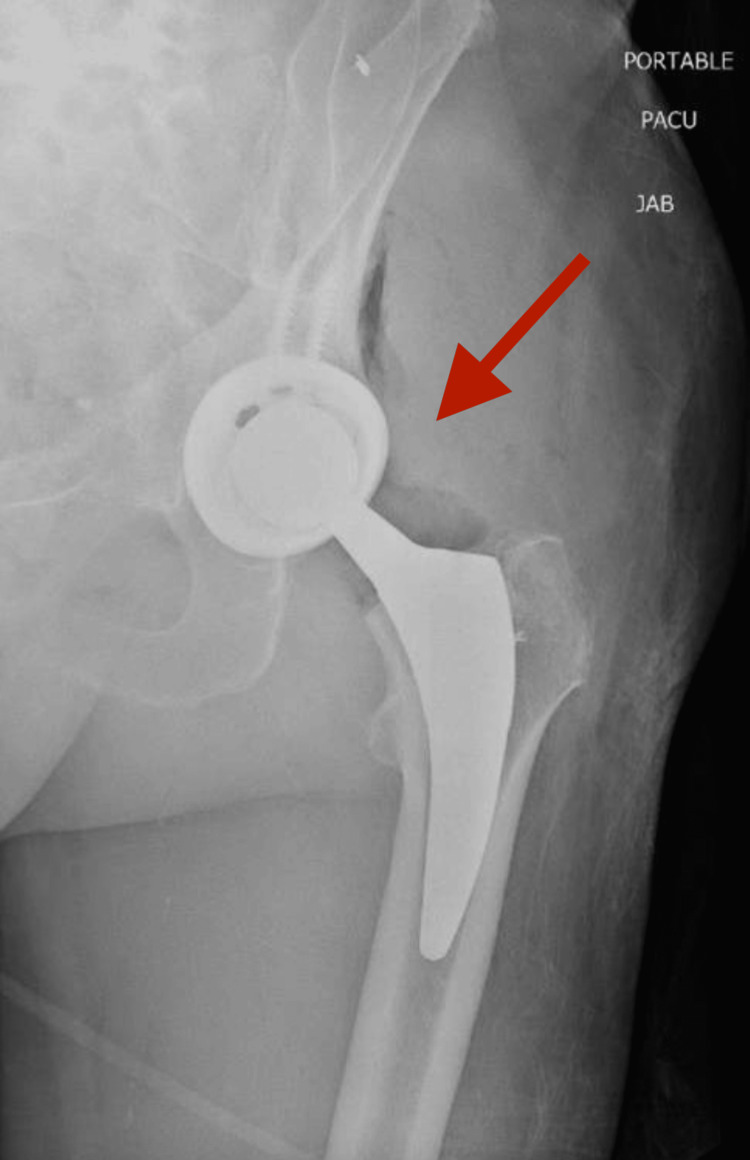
AP radiograph of left hip demonstrating concentric reduction of left THA AP, anterior-posterior; THA, total hip arthroplasty
